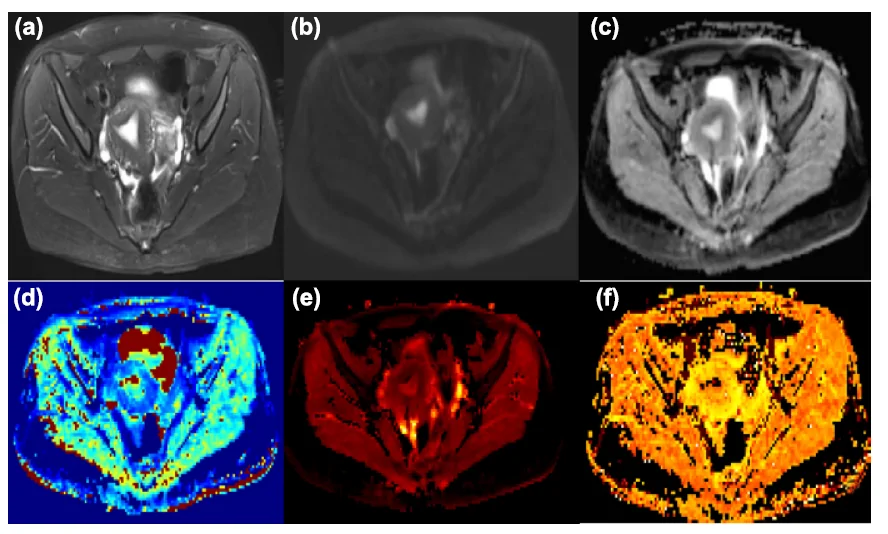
Normal endometrium with pseudo-color images of different fractional order calculus model (FROC) model parameters in a female patient aged 51 years. A-C. T2WI (a), DWI (b), and apparent diffusion coefficient (c) images were present. (d-f). The pseudo-color images of FROC model parameters β (d), parameter D (e), and parameter μ (f) were present.
